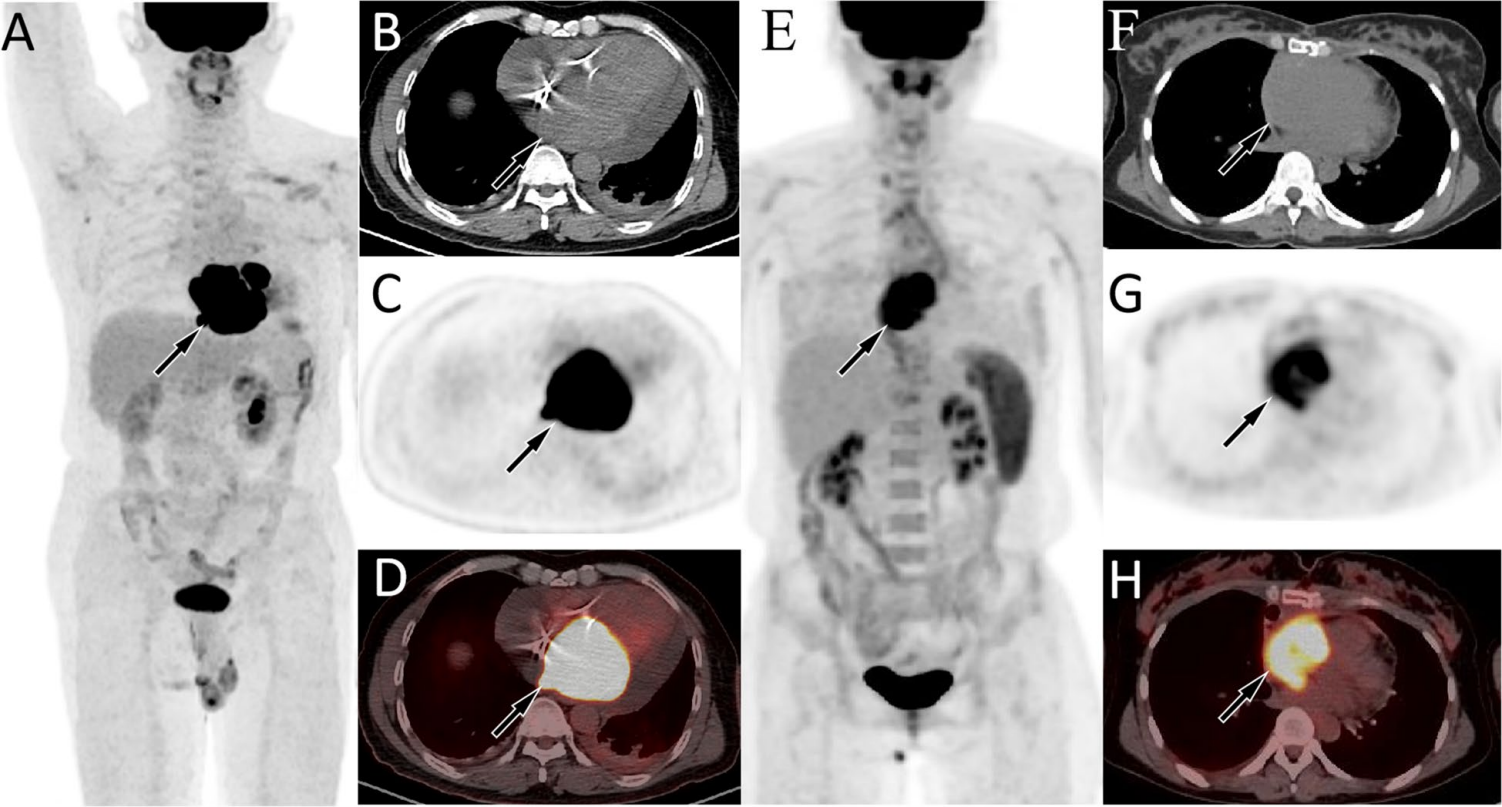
(A–D) A 67-year-old male patient with chest tightness and shortness of breath underwent echocardiography which revealed a left atrial mass, followed by PET/CT to assess its nature, and was subsequently confirmed as diffuse large B-cell lymphoma. The maximum intensity projection (MIP, A) showed a signifcantly increased ^18^F-FDG uptake in the heart region (arrow). Axial CT (B), PET (C) and PET/CT(D) showed that the lesion with high ^18^F-FDG uptake on the MIP map, which is isodense soft tissue mass located in the left atrium, with SUVmax, SUVmean, and MTV being 29.5, 15.49, and 67.52, respectively (arrows). (E–H) A 30-year-old woman with chest tightness and pain for 1 month underwent echocardiography and found a right atrial occupation, followed by PET/CT. The MIP map showed a lesion with significantly increased ^18^F-FDG uptake in the heart region (E, arrow). Axial CT (F), PET (G) and PET/CT (H) showed that the lesion was isodensity and located in the right atrium, with SUVmax, SUVmean, and MTV being 10.9, 6.4, and 46.29, respectively (arrows), which was later confirmed by pathology as angiosarcoma.
